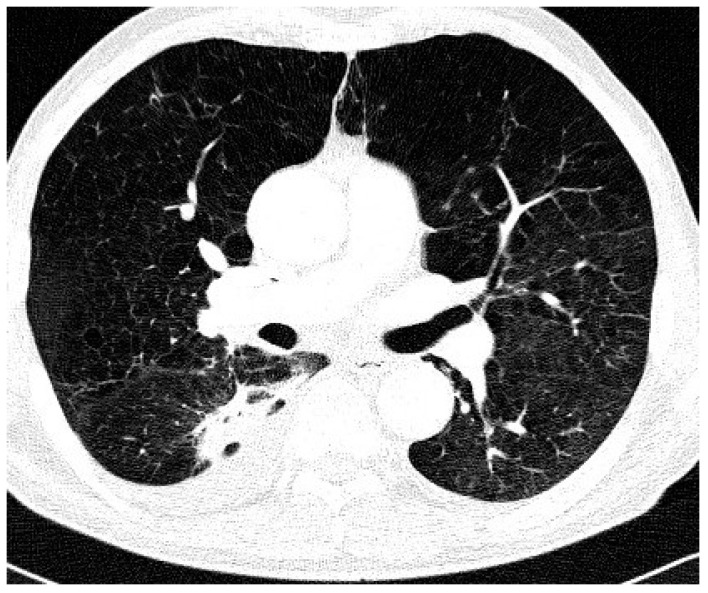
Trans-axial lung window CT scan in patient with NTM-LD and COPD shows diffuse emphysema and cavitating dense infiltrate and pleural thickening in the right apical segment of the lower lobe.
